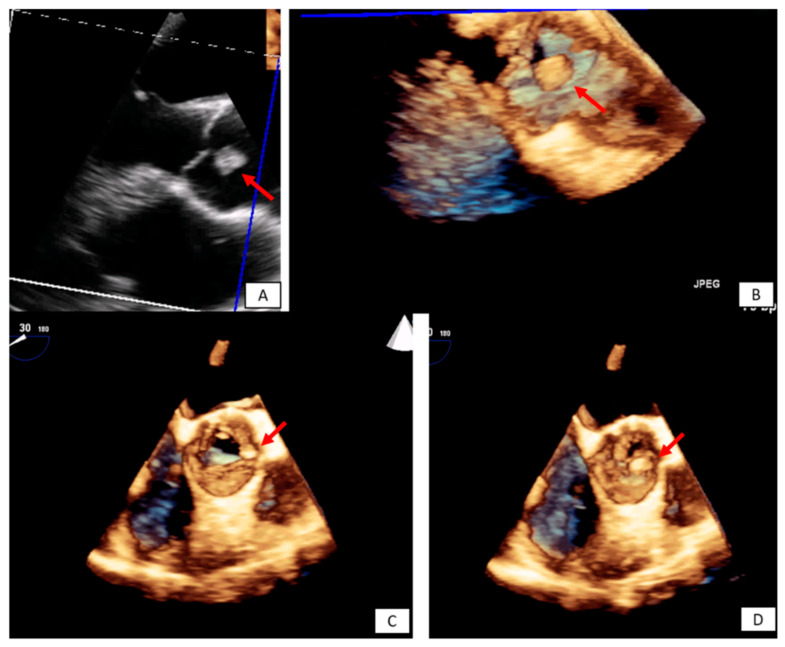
Incidental finding on TTE in an asymptomatic patient followed by TEE. The transesophageal image revealed a papillary fibroelastoma attached to the left cusp of the aortic valve (red arrows) 3D TEE with cropping in long axis (A,B), and short axis view ((C,D) during systole and diastole, respectively). Please note that the pedunculated lesion is attached on the tip of the aortic surface of the aortic valve, is not protruding in the LVOT during systole and doesn’t lead to valve destruction or insufficiency.
